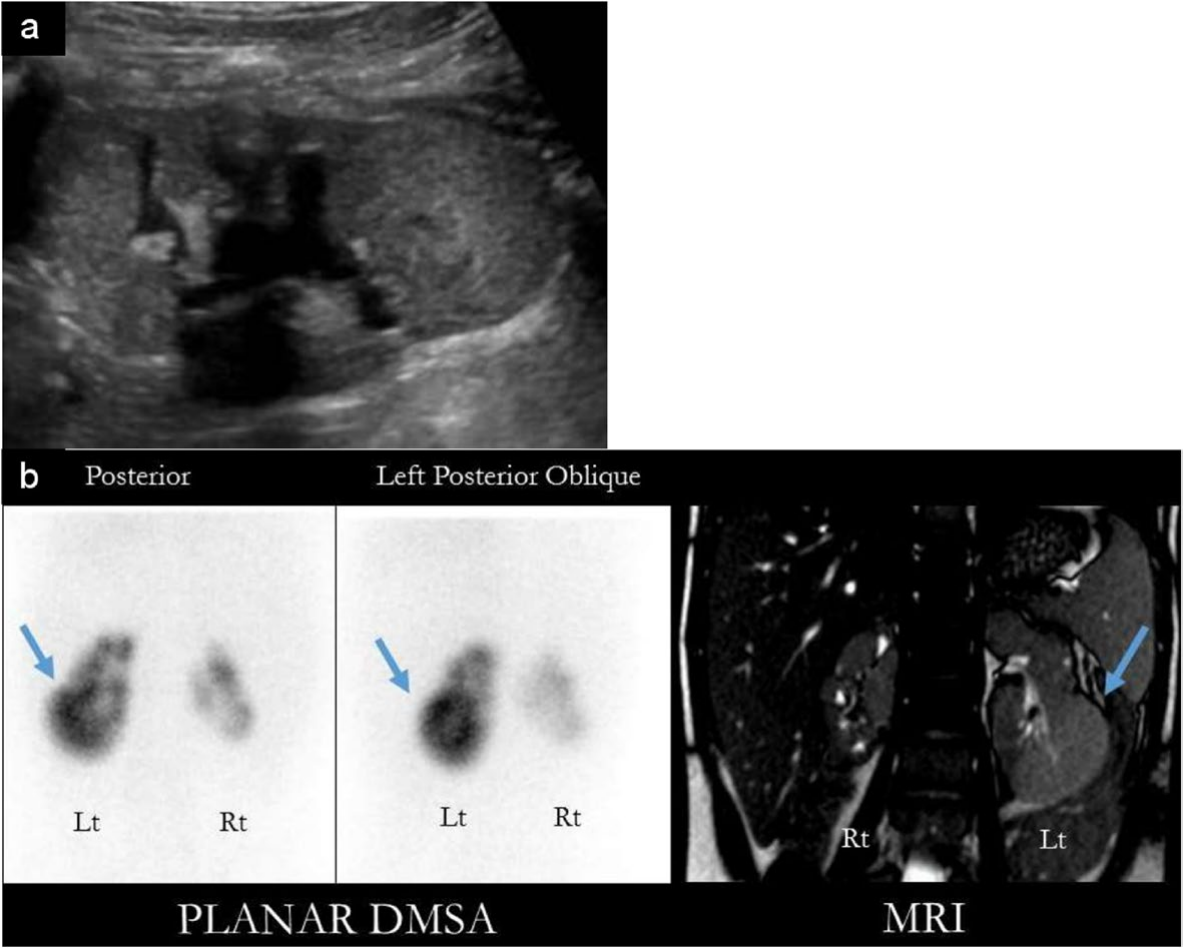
A US images showing a mass-like lesion in the lower pole of the left kidney. B DMSA study showing a FPT in the left lower pole corresponding to the site on MRI (blue arrow)
